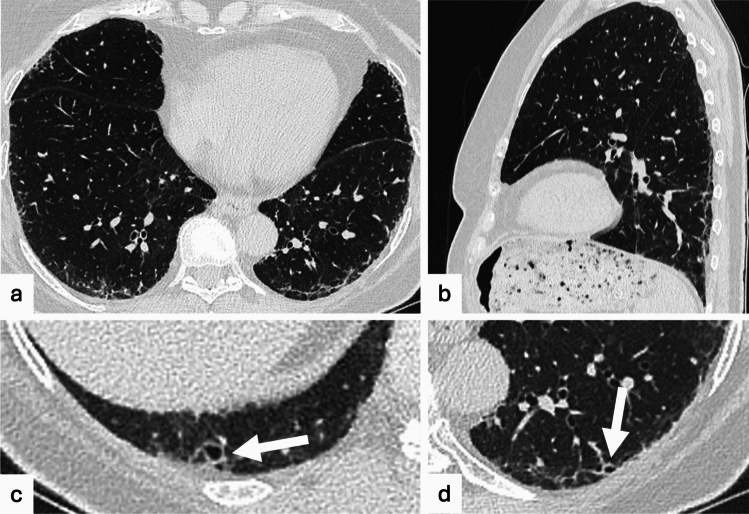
Seventy-one-year-old female. The chest CT scan, performed for a nodule seen on chest radiography, revealed bilateral peripheral reticulations with traction bronchiectasis (white arrows), classified as subpleural fibrotic ILAs
